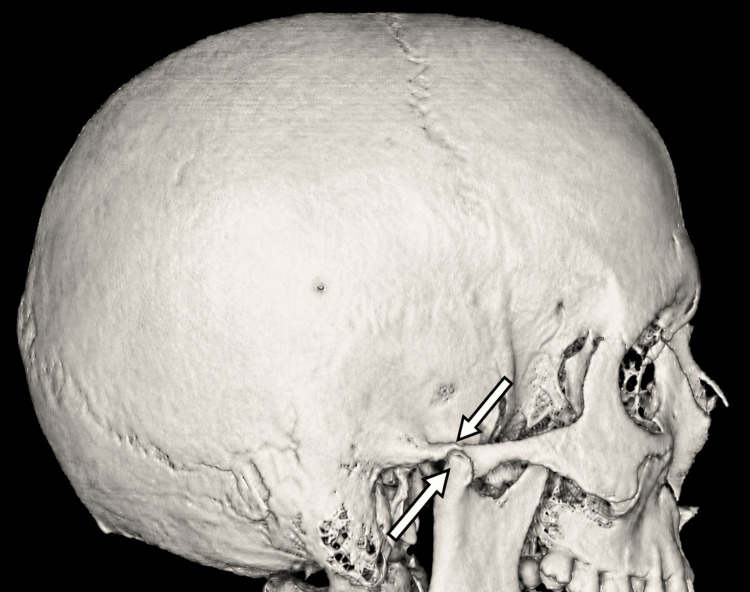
3D CT lateral volume-rendered image demonstrating a condylar fracture with superolateral displacement of the right TMJ relative to the zygomatic arch. The upper arrow indicates the superolaterally displaced right condylar fragment, and the lower arrow indicates the fracture line through the condylar head/neck region. CT, computed tomography; TMJ, temporomandibular joint
